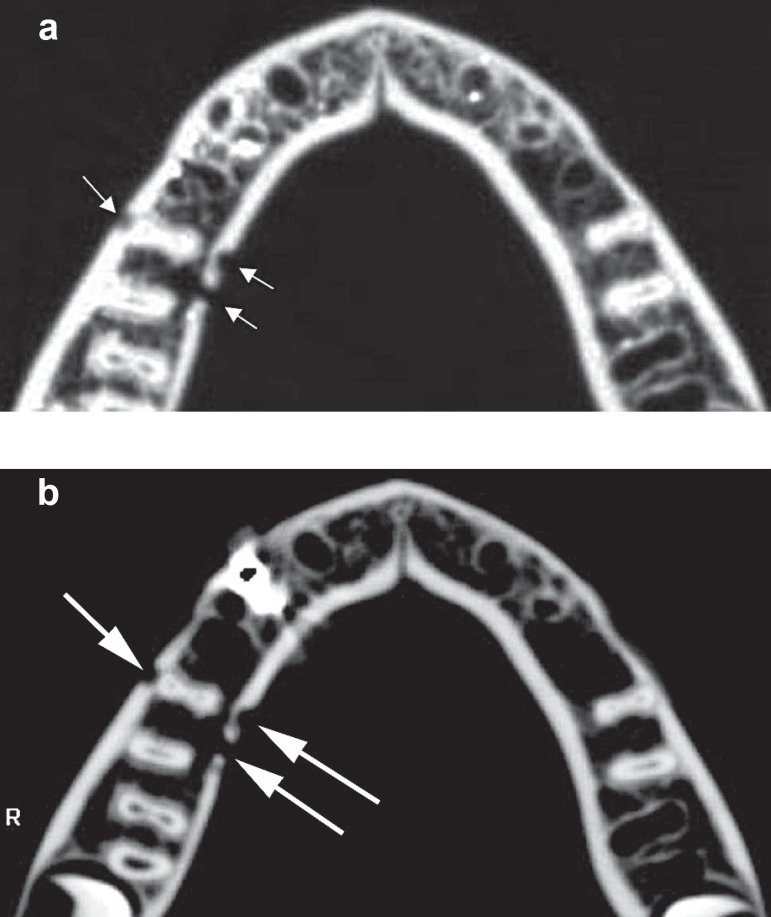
a) Axial CT of the mandible without metallic restorations and prostheses. The
